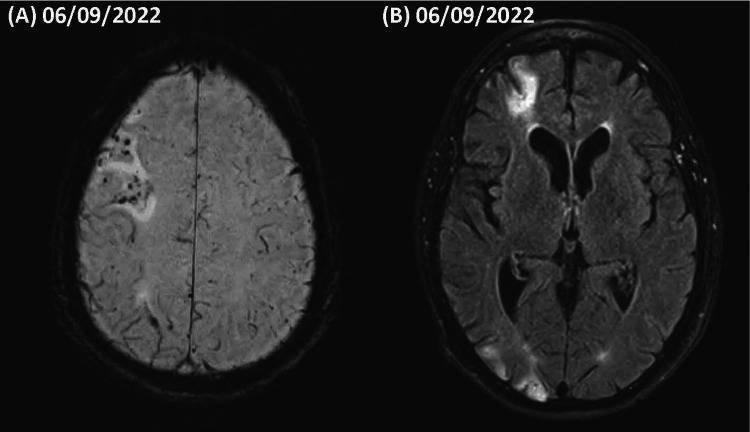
Brain MRI post-stroke (A) Susceptibility weighted sequence showing diffuse hemosiderin deposition and clustering in the right frontal lobe. (B) FLAIR sequence post aortic dissection with stroke. Note increased signal in the right frontal and occipital lobes.
